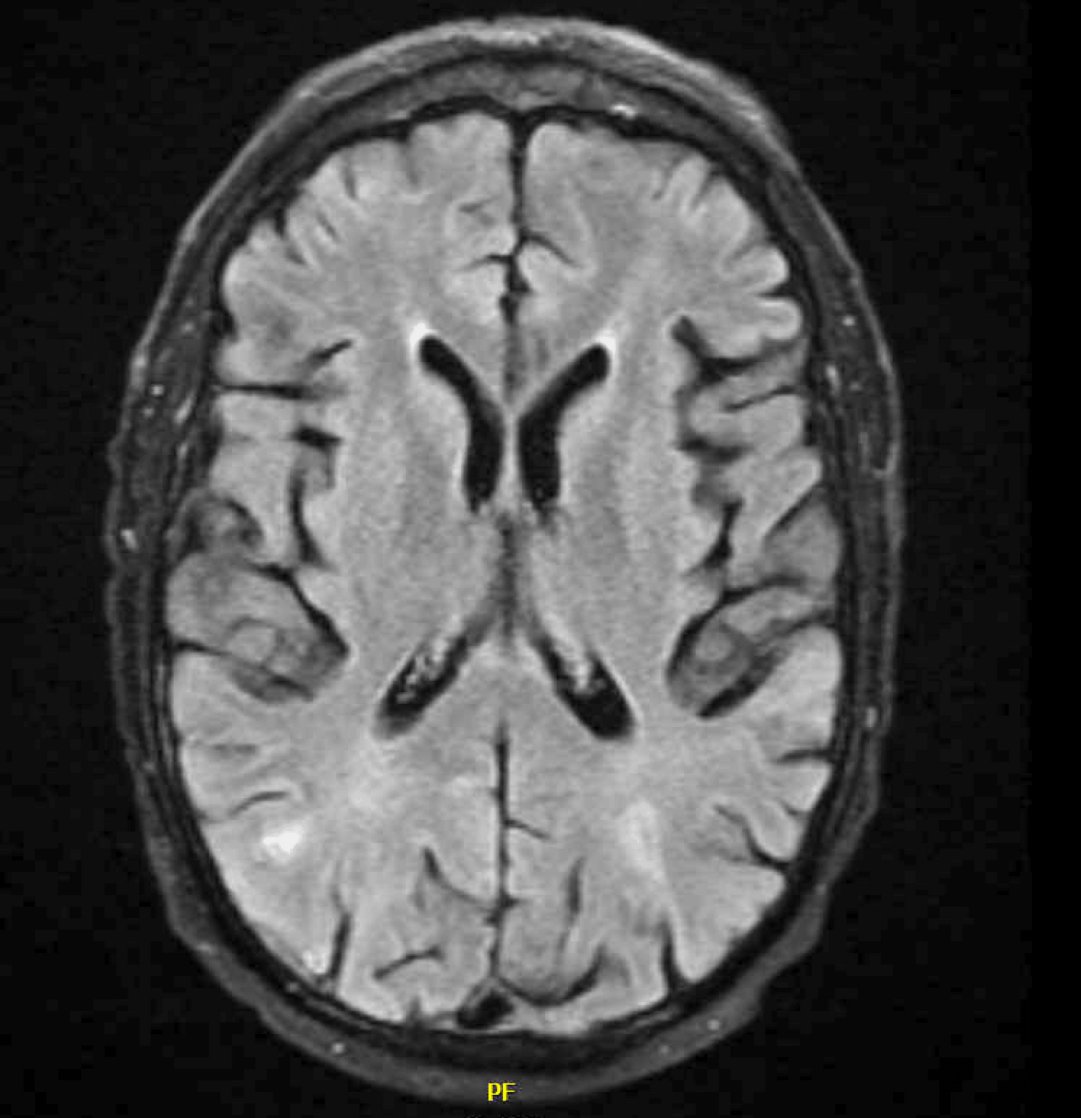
Repeat magnetic resonance imaging of the brain with a resolution of FLAIR hyperintensities FLAIR: fluid-attenuated inversion recovery
